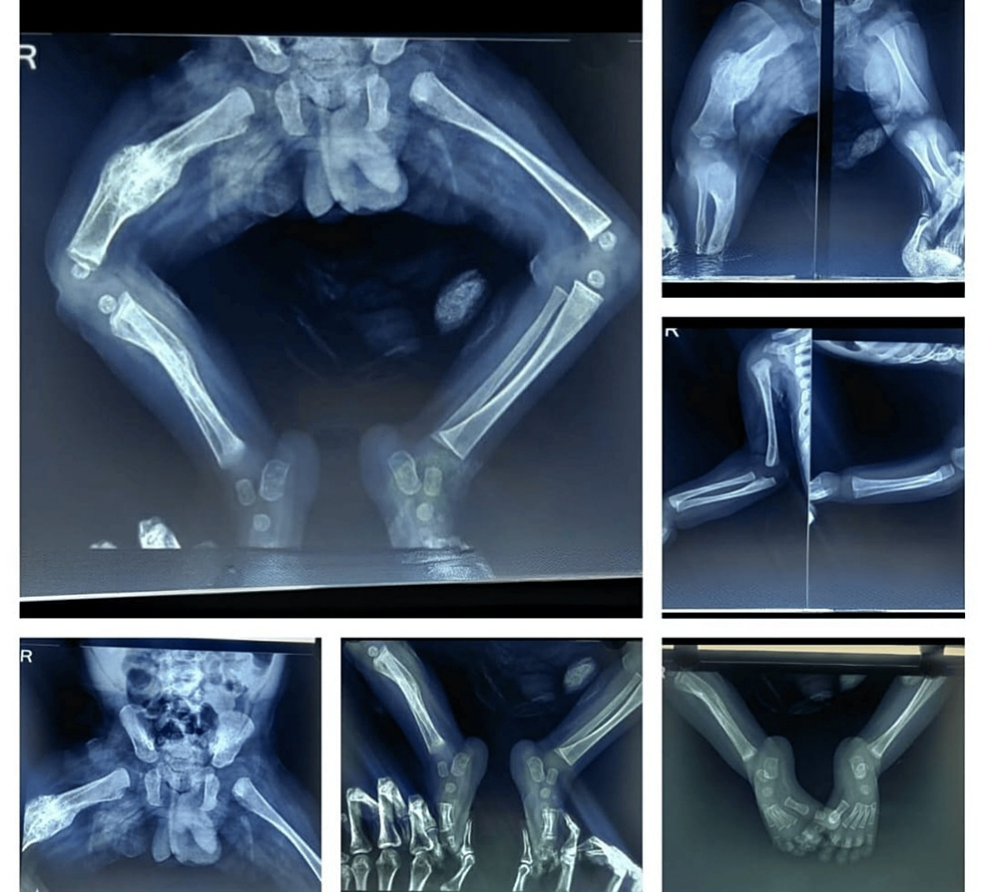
X-ray of the right femur with callus formation
